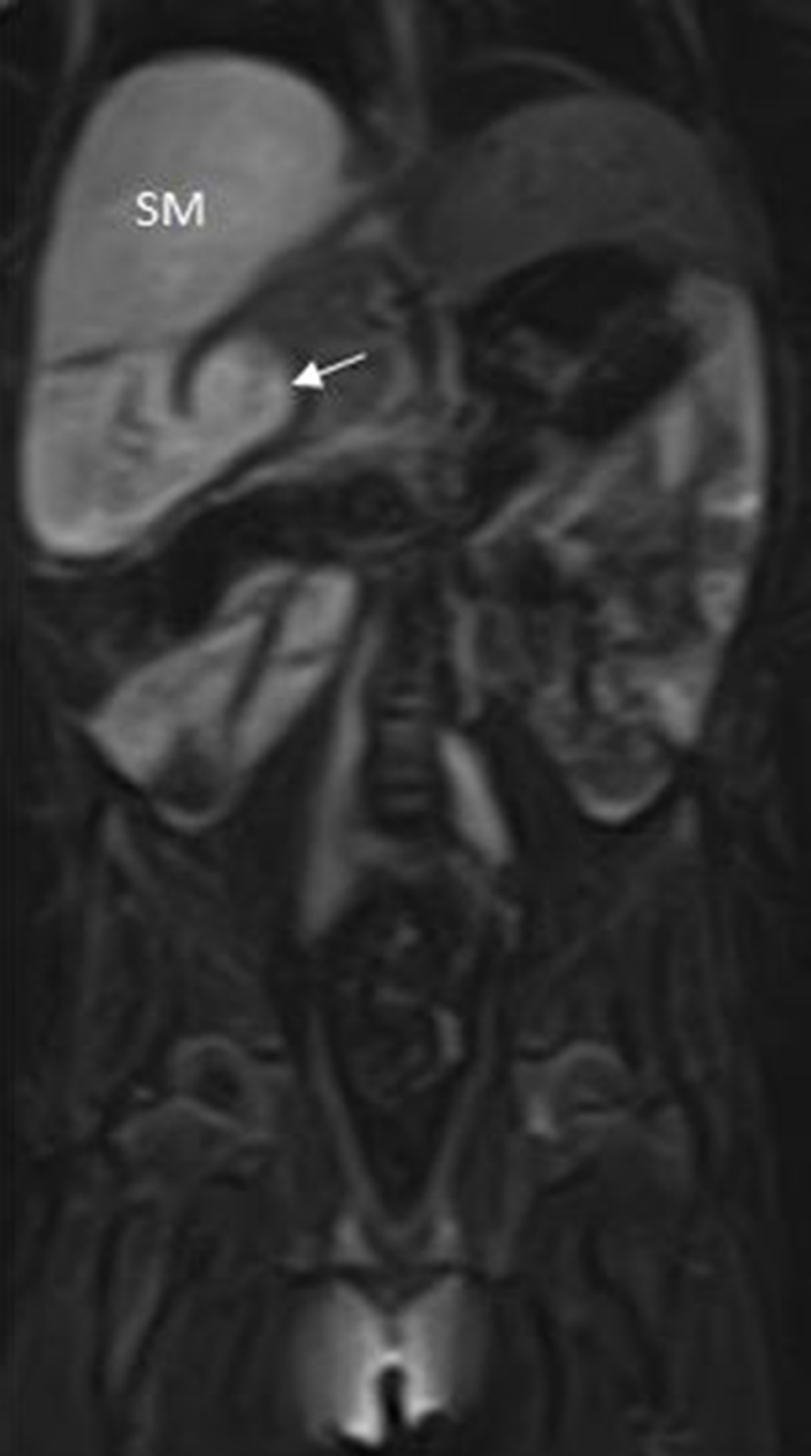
T*_2_ coronal MRI view showing fluid-filled SM with thicken pyloric wall (arrow). SM, stomach.
